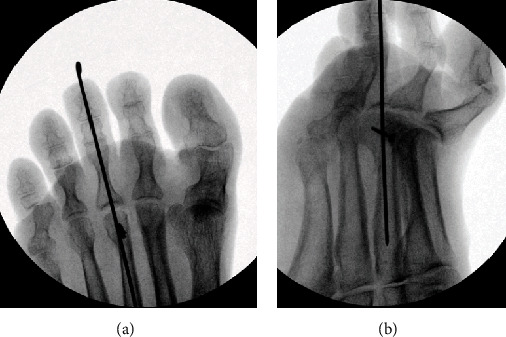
Intraoperative X-ray after realignment performing Weil's osteotomy, extensor brevis transfer, and temporary arthrodesis of the second toe.
